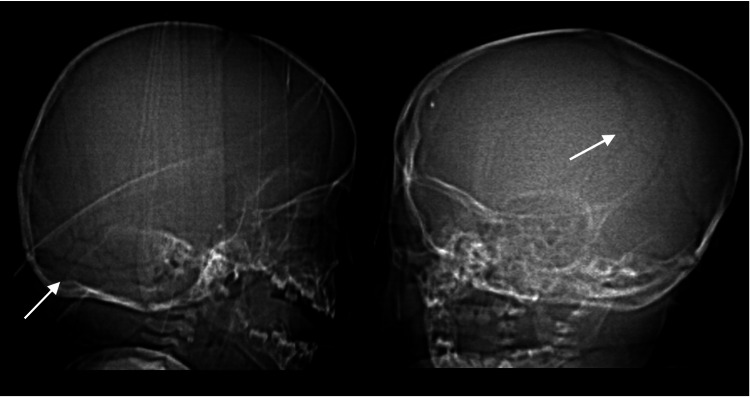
CT scan findings of a patient with accessory cranial suture in a 13-month-old male, sagittal (left) and coronal (right) views. A nondepressed fracture of the right occipital calvarium is present without associated soft tissue swelling.
